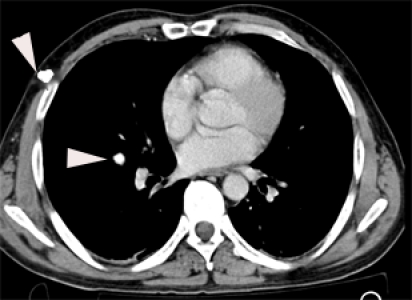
Thoracic CT. White arrows – metallic fragments (subcutaneous and middle lobe of the right lung).
